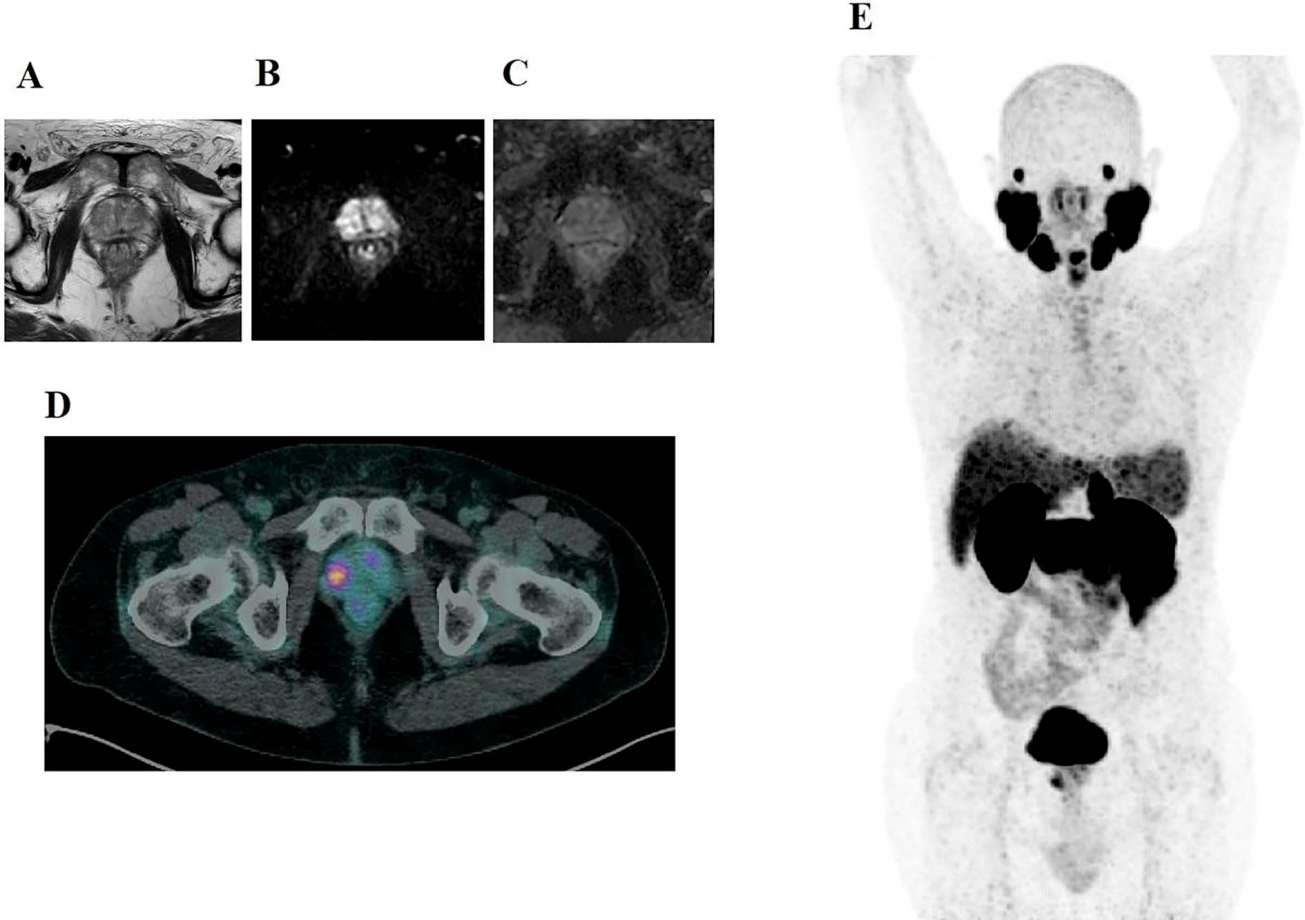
A 72-year-old man with a serum PSA of 4.5 ng/mL and a PSA density of 0.10 ng/mL/cc. BIOPSTAGE mpMRI was negative for suspicious findings (PI-RADS <3), while PSMA PET detected a focal uptake in the right anterior apex of the prostate. Axial T2-weighted MRI (A) shows no significant abnormalities. Axial diffusion-weighted imaging (DWI) (B) reveals no focal hyperintense signals, and the apparent diffusion coefficient (ADC) map (C) shows no restricted diffusion. In contrast, axial PSMA PET (D) and MIP PSMA PET (E) demonstrate focal uptake in the right anterior apex (SUVmax: 8.1; SUVratio: 3.6). Biopsy confirmed an ISUP 3 prostate cancer focus in the PSMA-positive region and minor ISUP 1 bilateral foci. This case highlights the complementary role of PSMA PET in detecting csPCa lesions missed by mpMRI.
